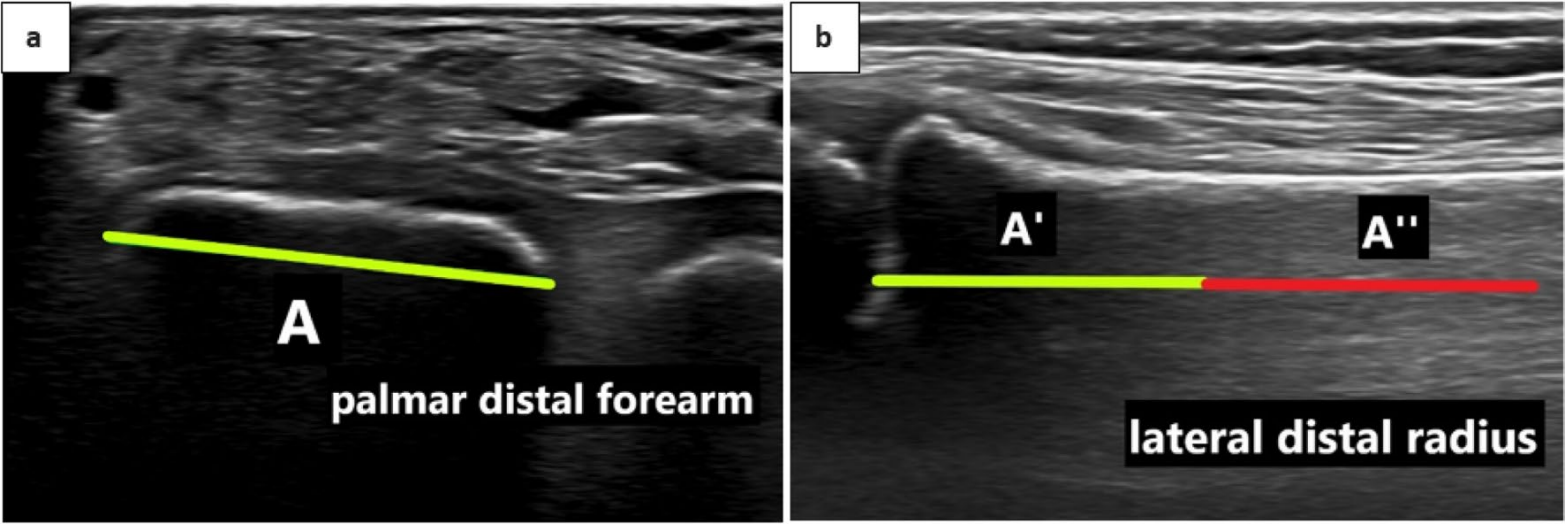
a Transverse ultrasound of the palmar distal forearm; A marks the width of the radius at the level immediately proximal to the growth plate which approximates the width of the radius growth plate; b Longitudinal ultrasound of the lateral distal radius; A’ marks the width of the radius growth plate as determined in the transverse ultrasound and is applied twice to determine the diametaphyseal junction zone A’’
